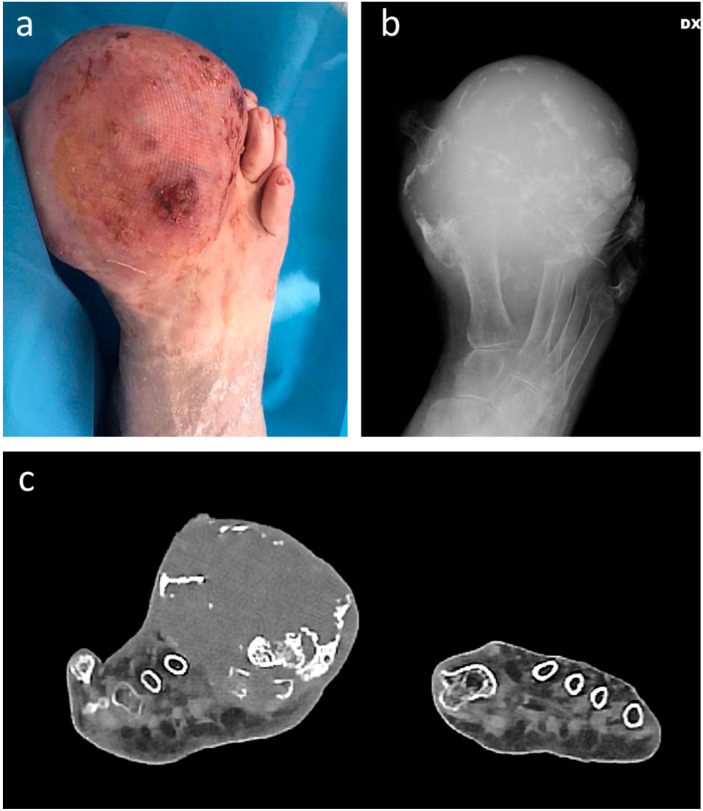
Clinical (a), plain radiograph (b), and coronal CT scan (c) appearance of gr. 2 chondrosarcoma in a 95-year-old female patient, appearing as swelling gradually increased over the last 15 years. A below-knee amputation was performed.
